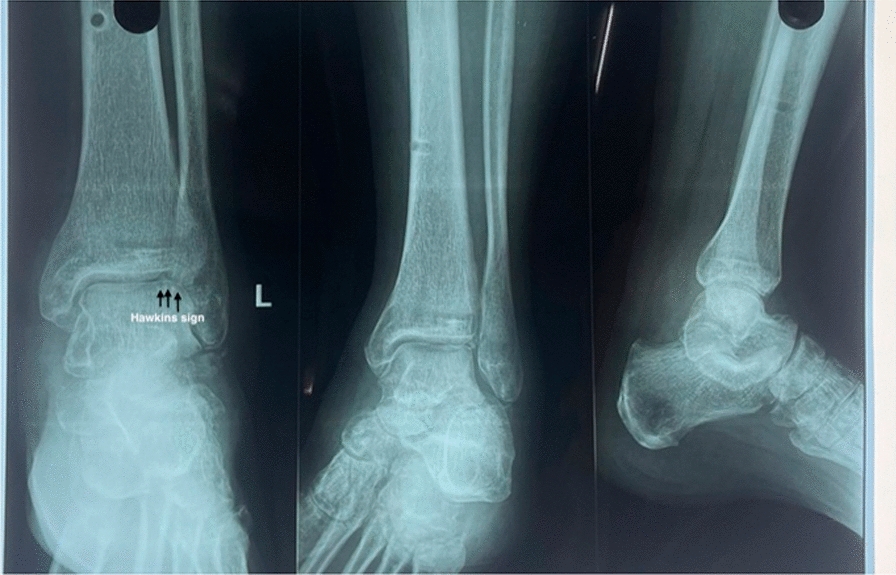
Radiograph 3 months post injury
